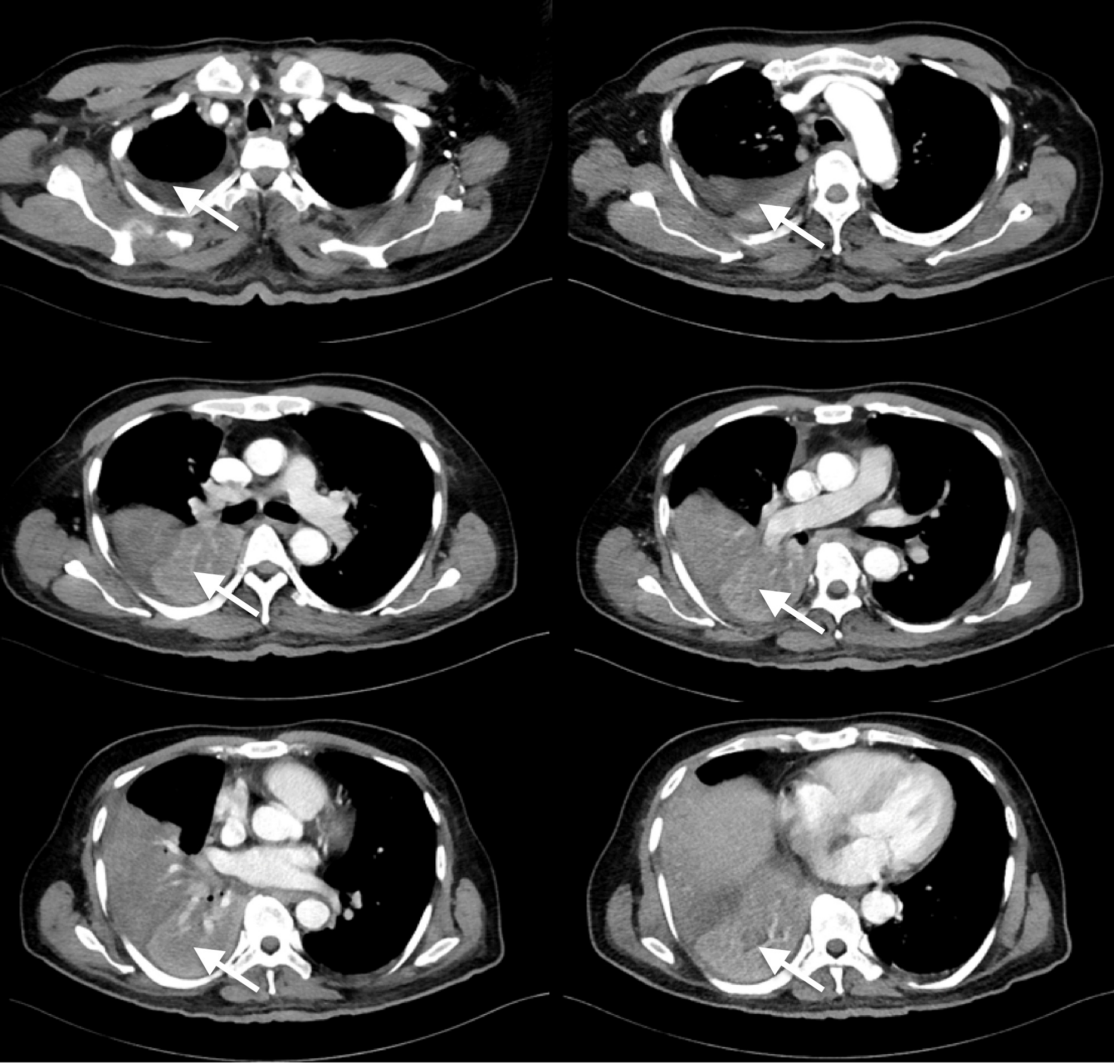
CT of the chest showing homogenously enhancing mass in the right bronchus intermedius causing collapse of the right lower lobe in addition to a small right-sided pleural effusion
